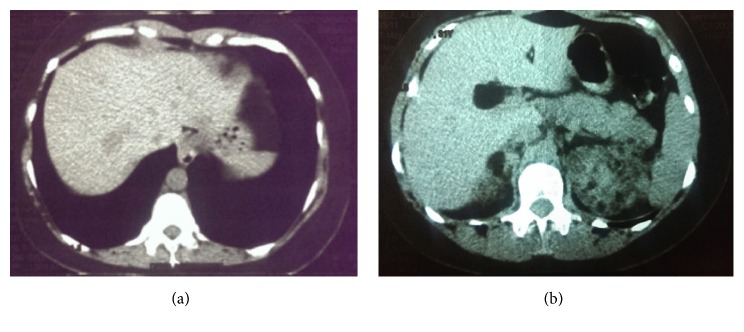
(a) CT cut from the abdomen showing multiple hypodense lesions in the liver with the largest lesion in the right lobe. (b) Lower cut showing multiple angiomyolipomas in the upper pole of the right and left kidney, which have interrupted the normal size, shape, and density of the organ. The Hounsfield values of the lesions are similar to that of the subdermal fat tissue.
